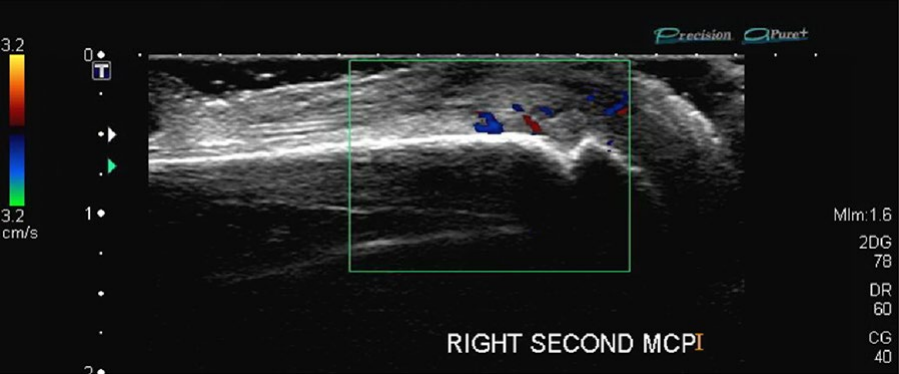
Color* Doppler imaging demonstrating a minimal effusion but marked synovial proliferation with moderate Doppler flow
